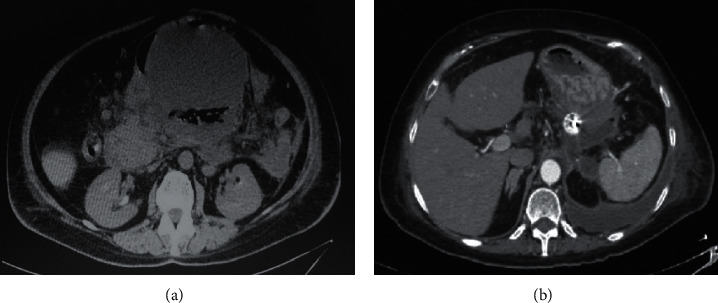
Abdominal CT scan showing (a) a huge pancreatic pseudocyst with air and fluid content and (b) resolution of most of the pseudocysts after AXIOS drainage.
